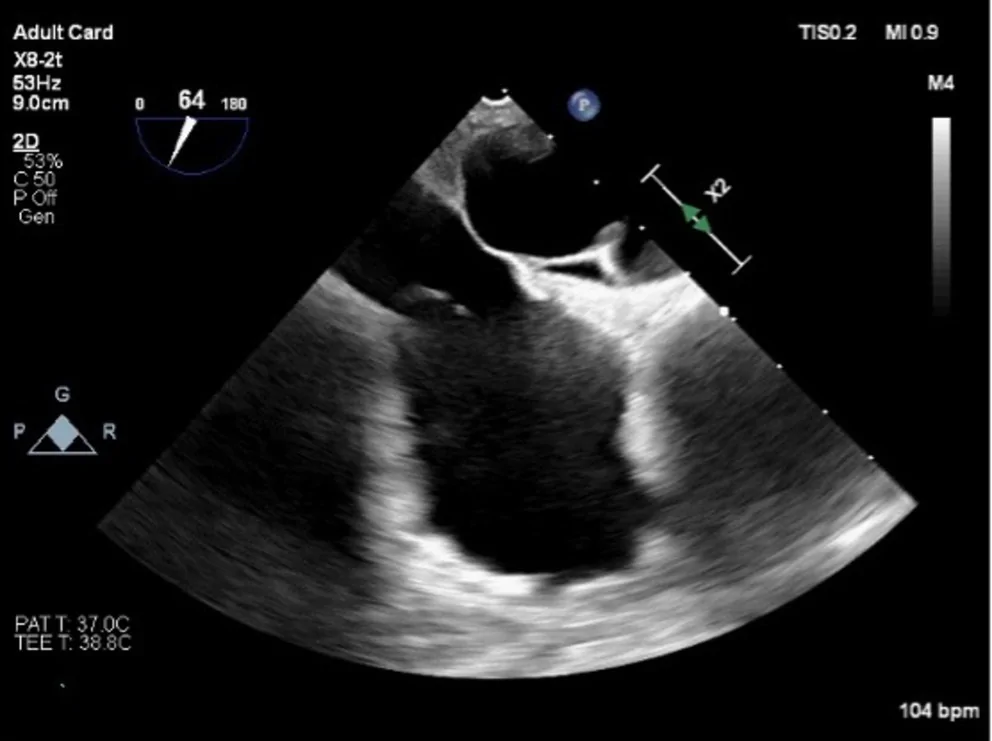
During transoesophageal echocardiography, there was an incomplete crescent‐like membrane at the left atrial septum causing an incomplete separation of the left atrium, findings indicative of Cor triatriatum sinister, and a prominent venous valve remnant such as Eustachian valve.
